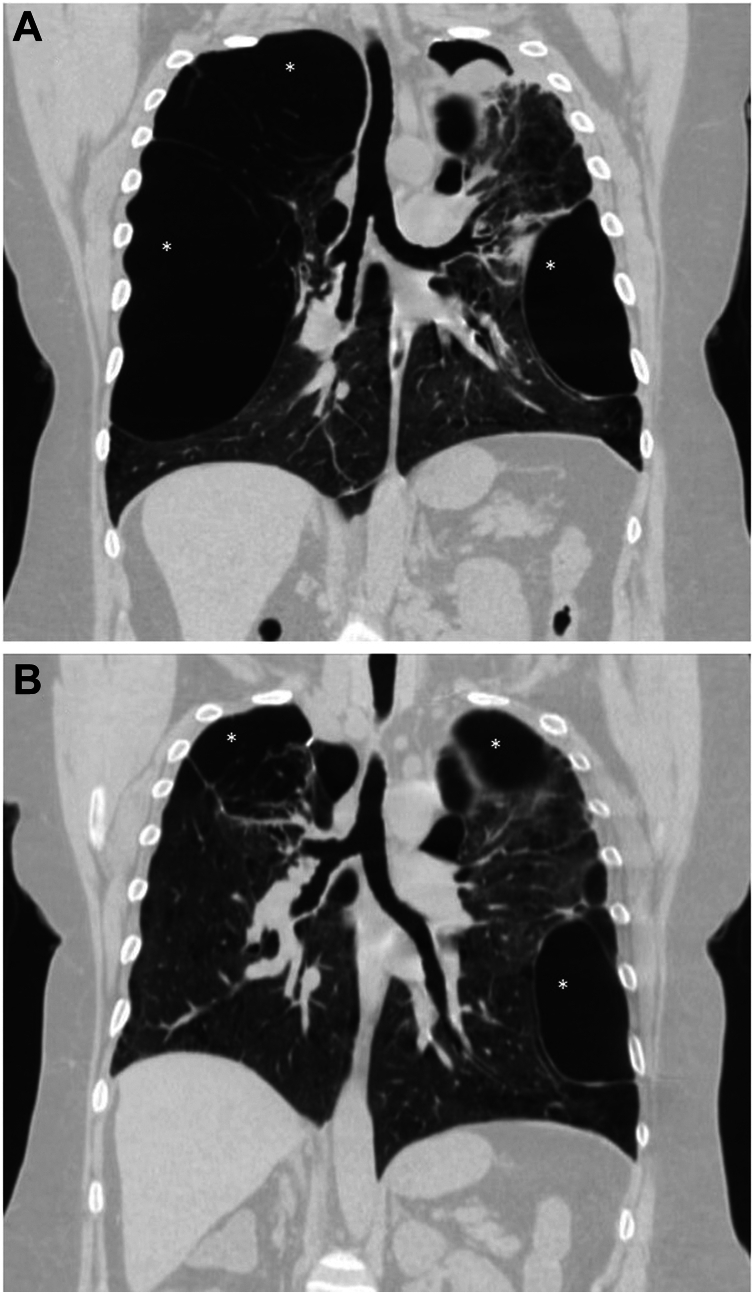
A, B, Prebullectomy and postbullectomy imaging. A, CT imaging of the patient before bullectomy, indicating extensive bullae, with the right side more affected than the left (bullae denoted by asterisks). B, Postbullectomy CT imaging, showing improvement of air trapping in the right lung, evidenced by decreased right lung volume. Bullae persist on the left side (bullae denoted by asterisks).
